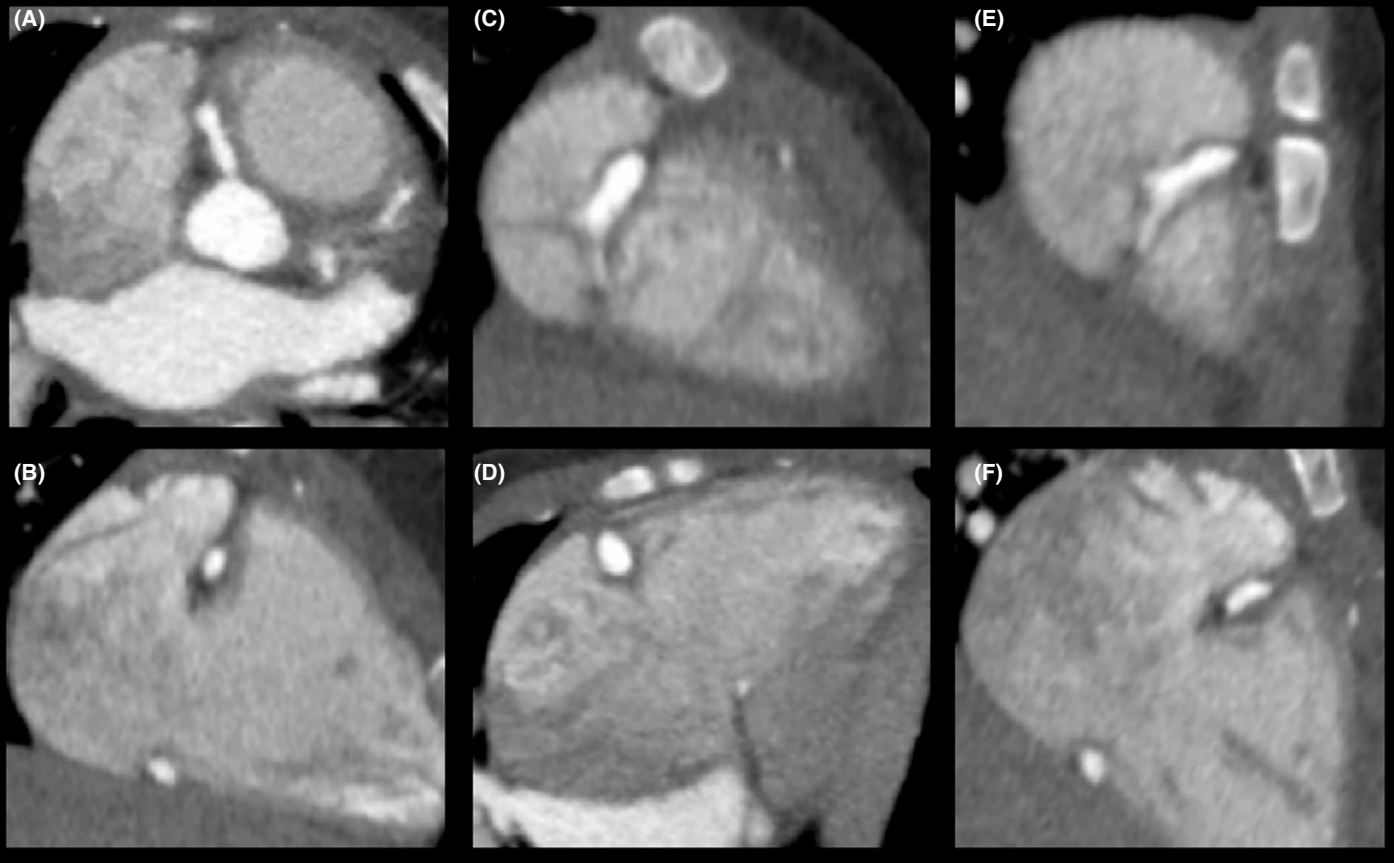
Contrast‐enhanced CT angiographic images of the RCA, demonstrating three regions of aneurysmal dilatation. Proximal RCA with aneurysmal dilatation in the perpendicular short‐axis view (A) and in the long‐axis view (B), in total measuring 4.3 × 2.9 × 9.8 mm. The short axis (C) and the long axis (D) of the aneurysmal dilatation of the RCA at the origin of the acute marginal artery, measuring 6.4 × 4.8 × 10 mm. Likewise, in the short axis (E) and the long axis (F), it is depicted at the bifurcation of the PDA and the atrioventricular nodal artery, measuring 4.5 × 3.2 × 11 mm
